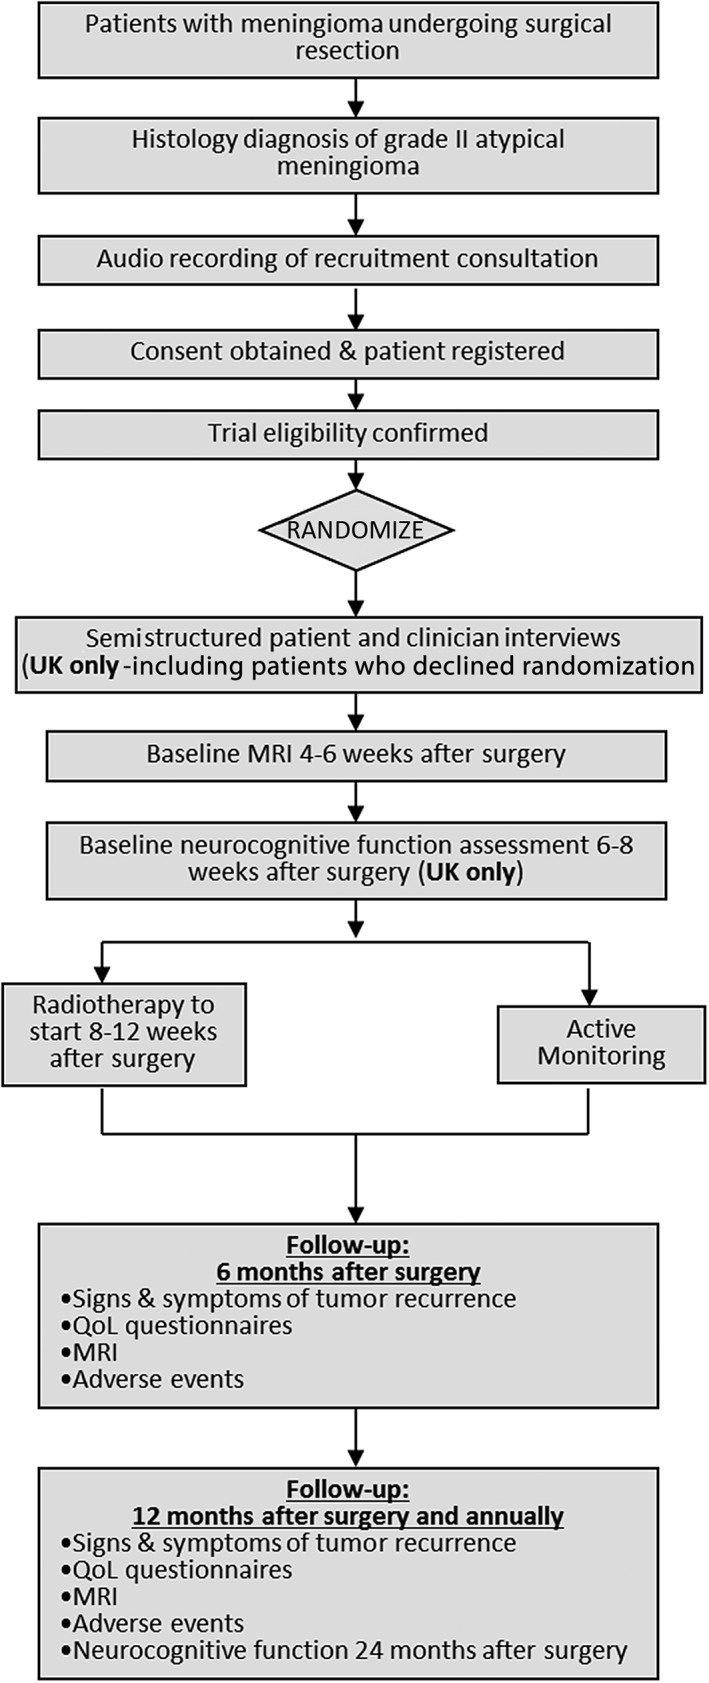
Summary of the design of ROAM/EORTC‐1308 and the embedded qualitative study.Abbreviations: MRI, magnetic resonance imaging; QoL, quality of life.
